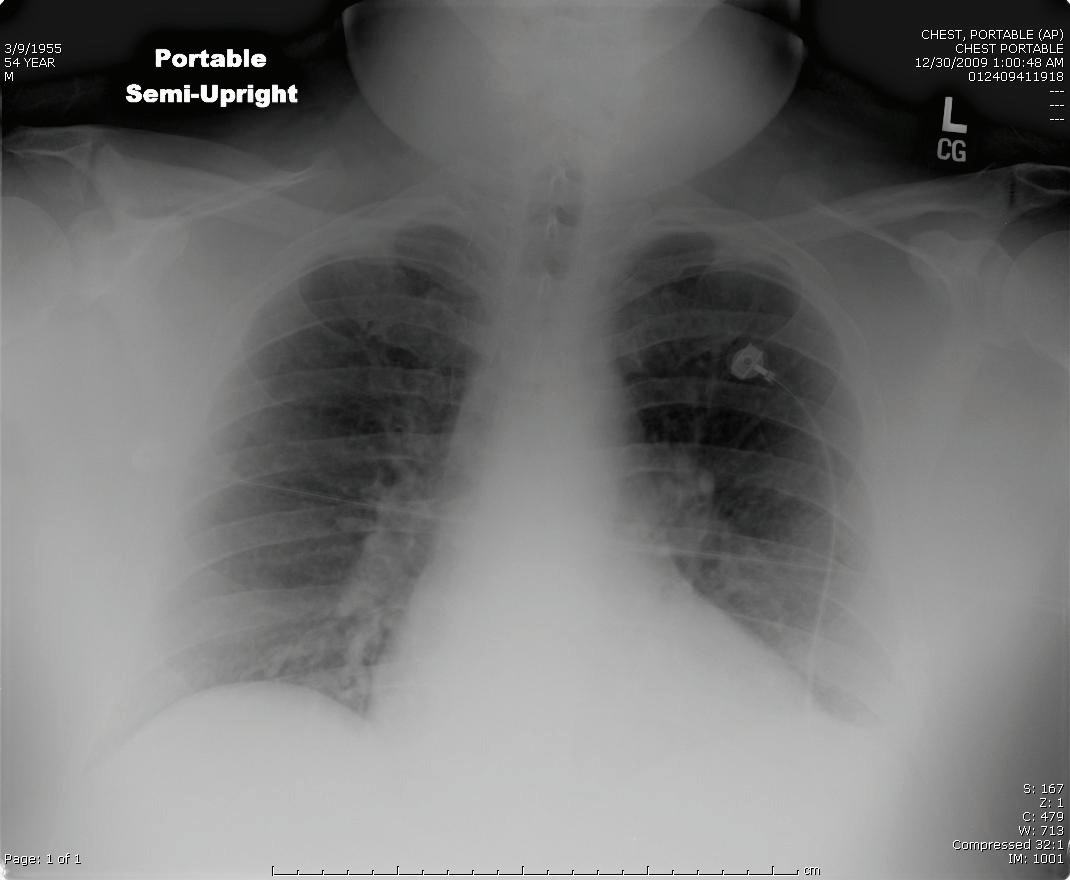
Upright portable chest X-ray. No acute cardiopulmonary process was noted and no intra-abdominal free air.
